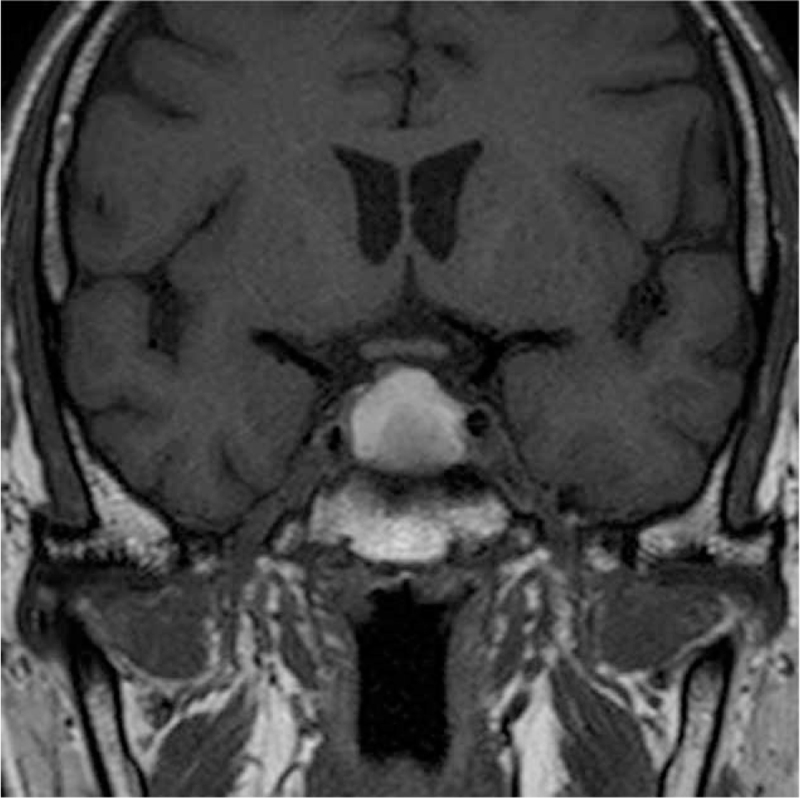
Coronal view of T1-weighted sellar MRI: a pituitary macroadenoma, 2 cm in height, with both intrasellar and suprasellar components. MRI = magnetic resonance imaging.
